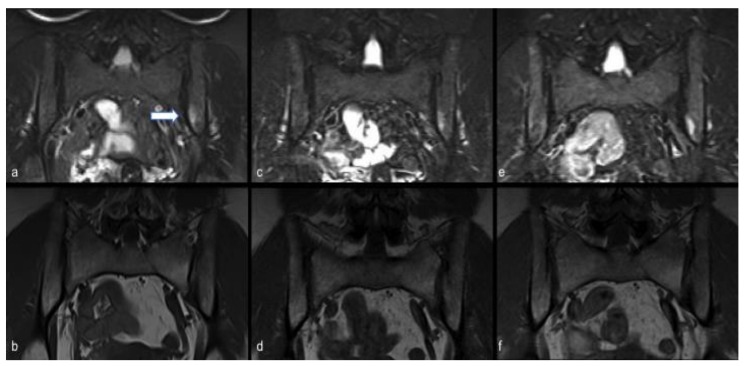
Case report 2. Persistent MRI findings of unilateral active sacroiliitis in a 53-year-old woman with lower back pain. At the time of first MRI examination, subchondral bone marrow oedema at the iliac side of the left sacroiliac joint was observed, appearing as band-like hyperintensity on short-tau-inversion recovery STIR T2-weighted semi-coronal image (arrow in (a)) with corresponding hypointensity on turbo-spin-echo TSE T1-weighted semi-coronal image (b). Oedema was found to persist at subsequent MRI examinations performed after one month (c,d) and six months (e,f).
